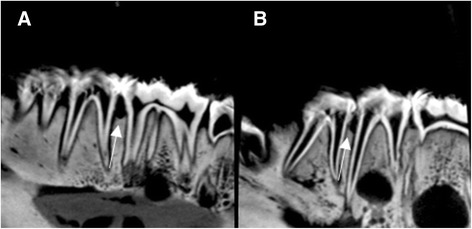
Sagittal sections of CBCT images. a. Crater formed after 6 h of acid exposure between second premolar and first molar. b. Vertical defect formed after 6 h of acid exposure between first and second premolar
